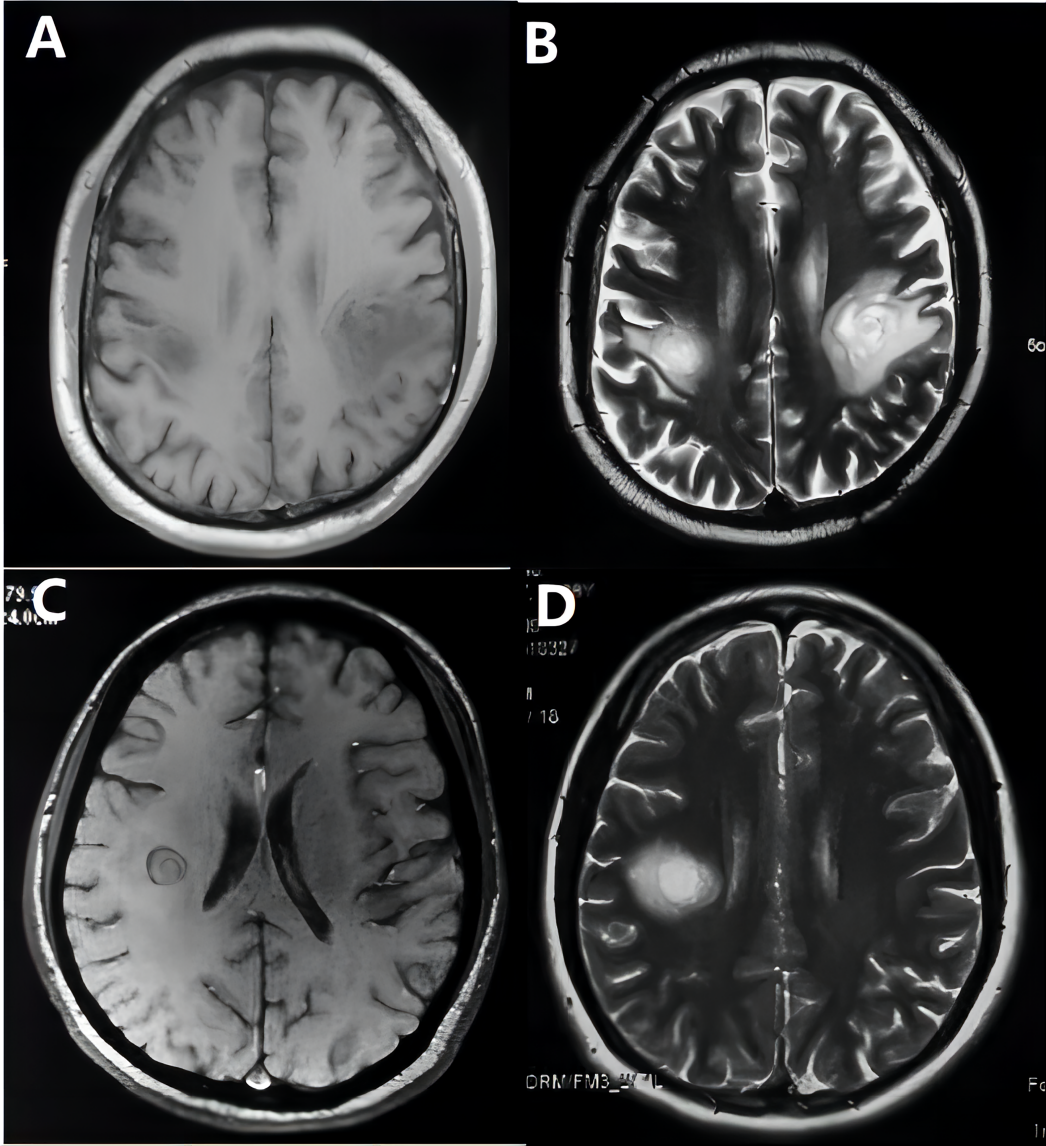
Balo images on T1-weighted (A, C) and T2-weighted (B, D) MRI of two patients.
